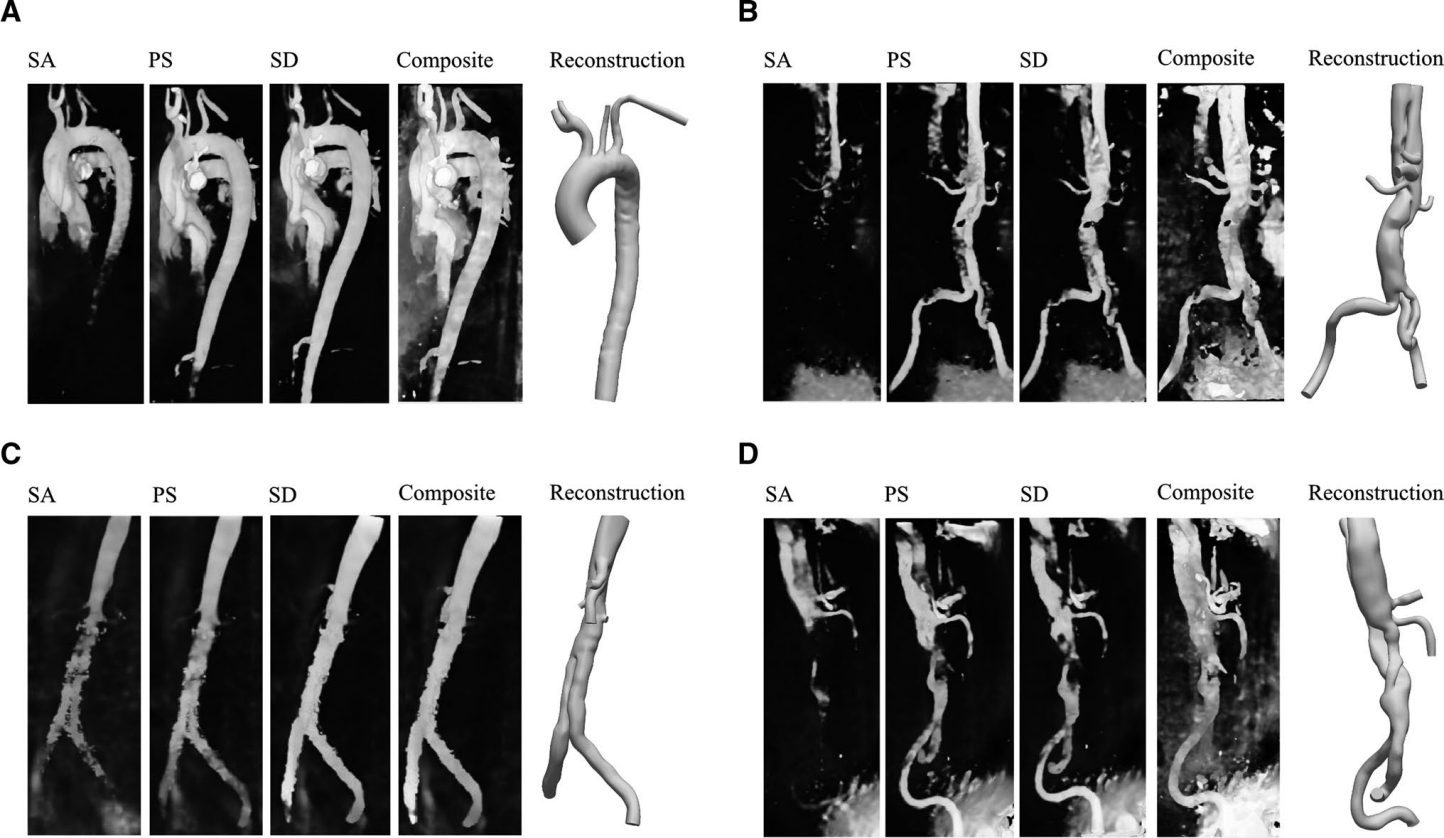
3D greyscale render of the velocity-derived contrast at SA, PS, and SD, the 4D Flow-MRI CPC-MRA, and the final SimVascular reconstruction for the A healthy volunteer, B patient 1, C patient 2, and D patient 3
